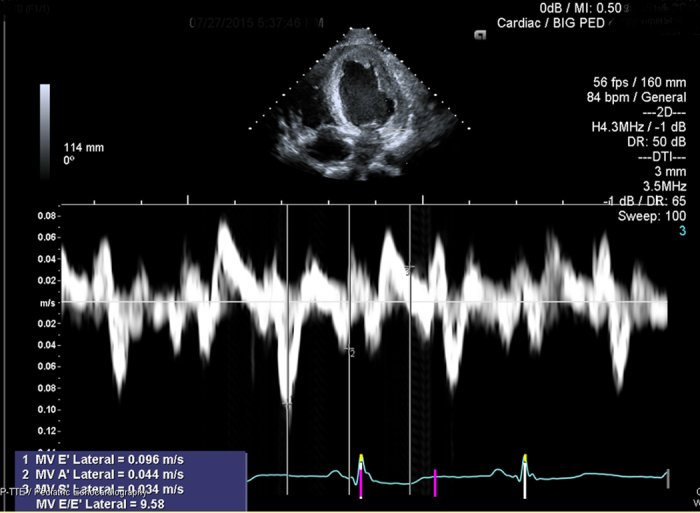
Tissue Doppler velocity at lateral mitral annulus of left ventricle.
